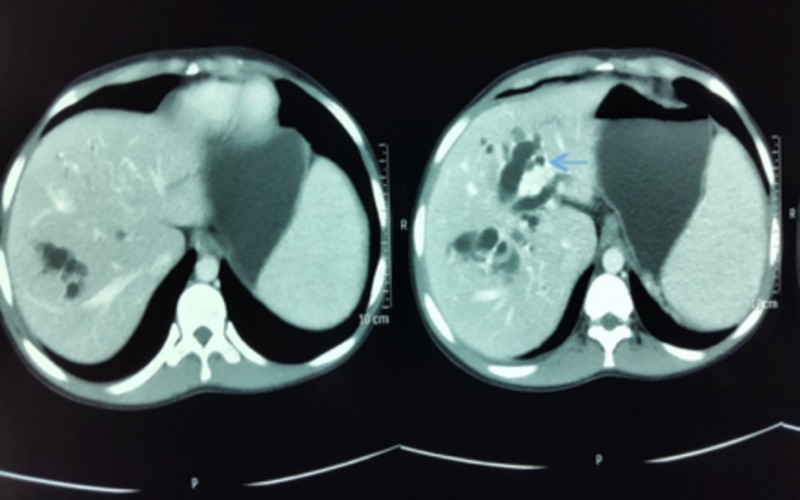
Intra hepatic biliary duct was grossly dilated and cystic (blue arrow) as seen in the computed tomography of the abdomen
